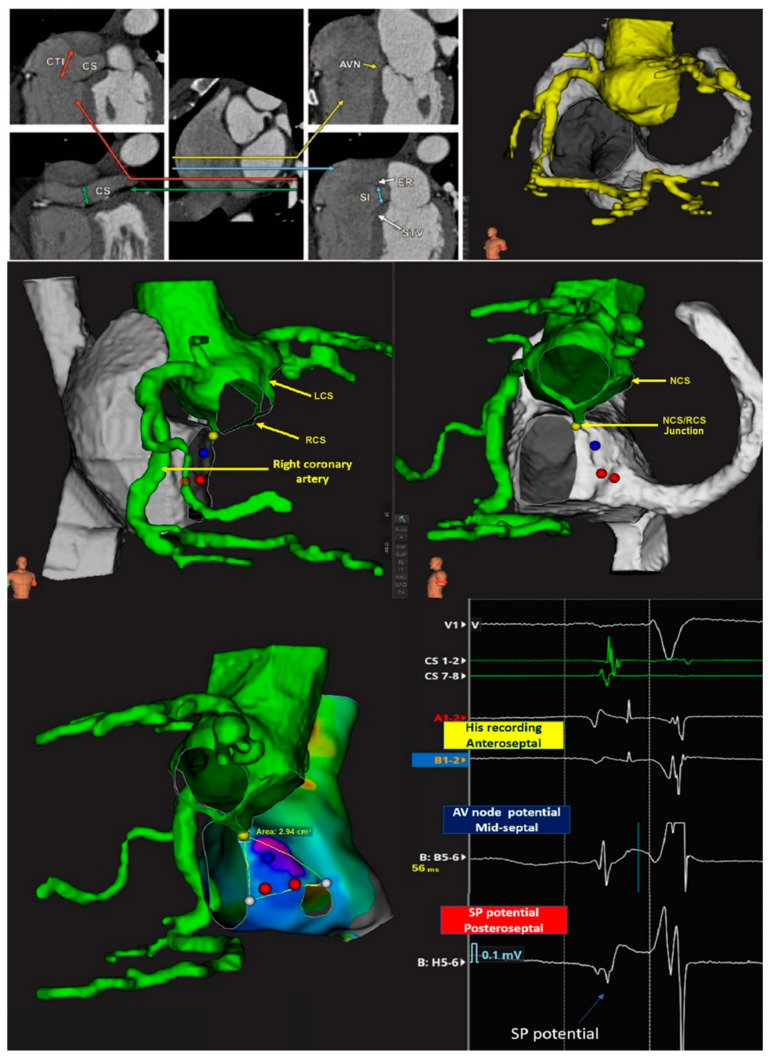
Merged acquisition from 3D mapping and reconstruction of computed tomographic datasets: step per reconstruction. Once the RA Rhythmia™ map has been acquired using Orion catheter, we aligned it with the reconstructed segmentations. This alignment allows us to clearly identify the aortic root relative to Koch’s pyramid. Lower panels: The position of the non-branching atrioventricular bundle inside the His cloud was identified at the site where the infero-septal recess of the aortic root meets the apex of the pyramid of Koch. The inferior right panel shows the electrograms recorded in Koch’s triangle. CTI = cavo-tricuspid isthmus, CS = coronary sinus, AVN = atrioventricular node, LCS = left coronary sinus, RCS = right coronary sinus, NCS = non-coronary sinus.
